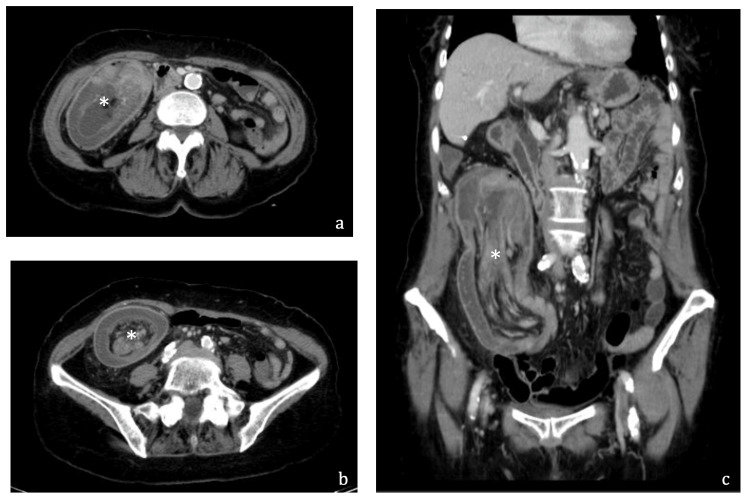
Abdominal and pelvic CT scan in transverse (a, b) and sagittal (c) views demonstrating intussusception of the right colon (asterisk)
